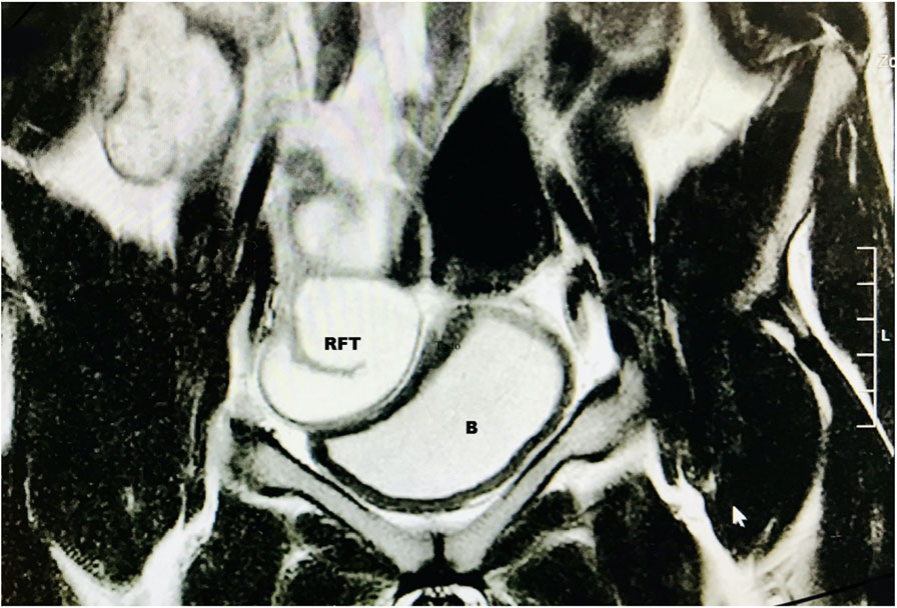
Coronal scan of pelvic MRI showing hydrosalpinx in case 1. B = bladder; RFT = right fallopian tube (C-shaped dilated tubular structure)
